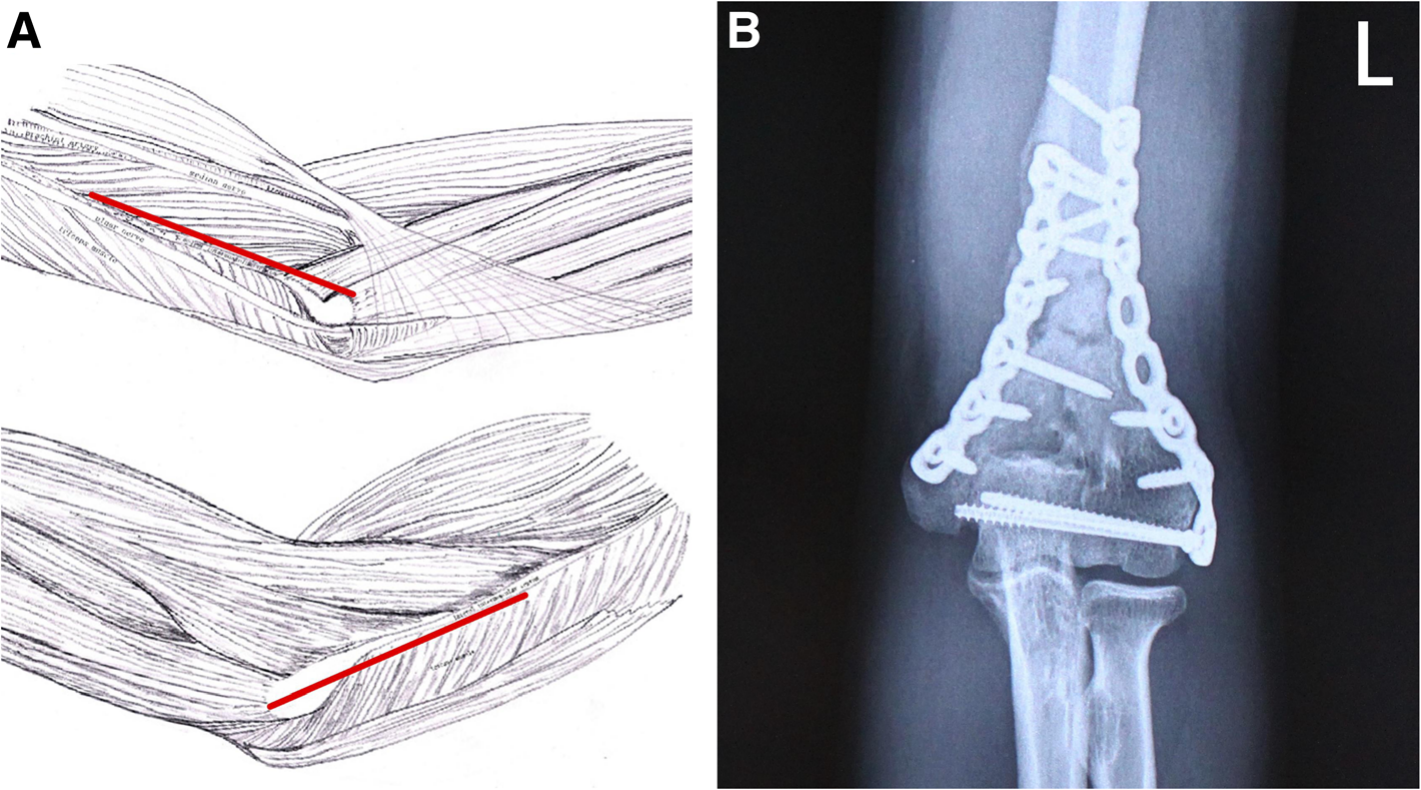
a The combined medial and lateral approach. The upper figure shows the ulnar side view, and the lower the radial side view. b The X-ray of the novel plating (combined anteromedial and anterolateral plating), with an extra lag screw
